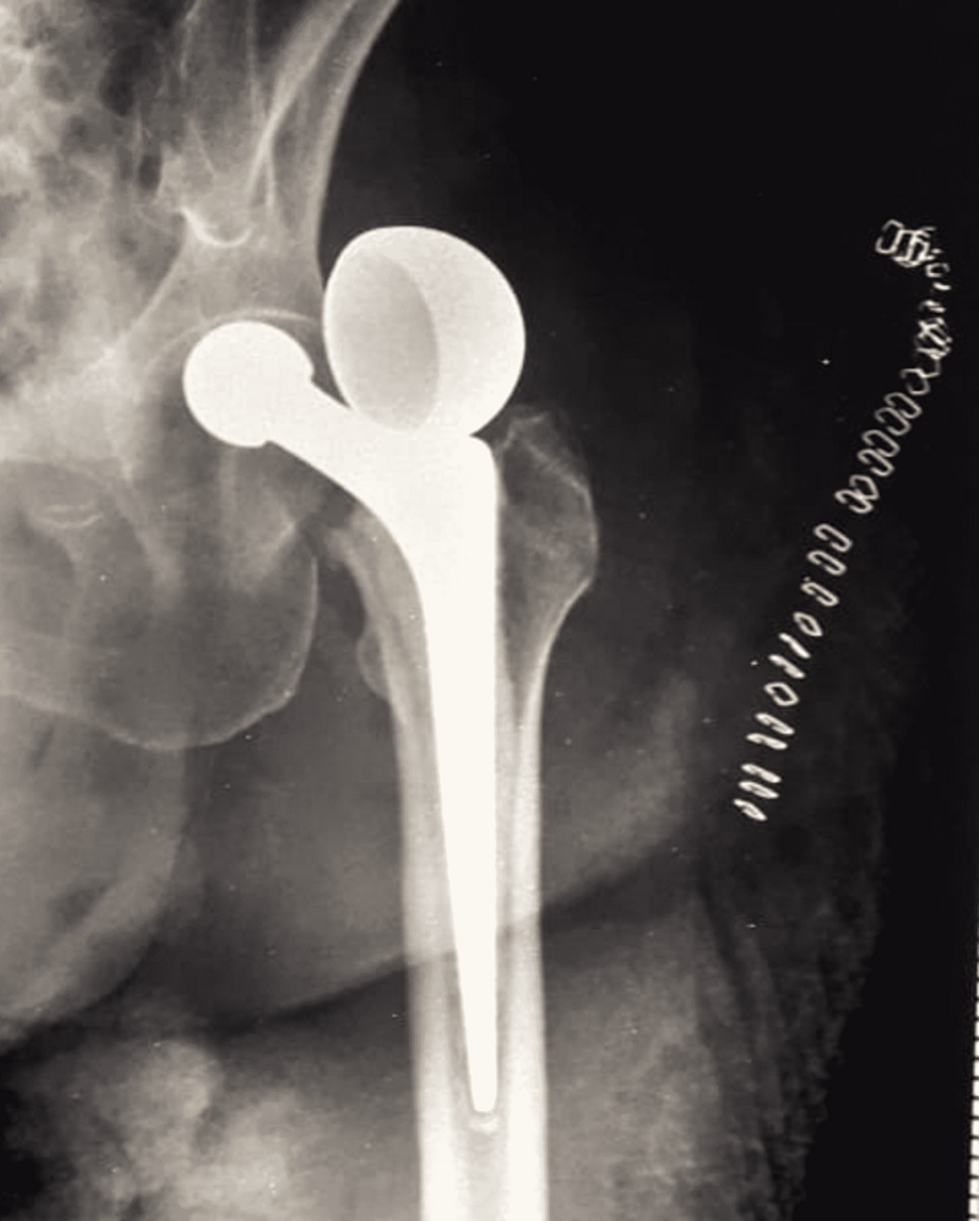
X-ray of the left hip showing dislocation and uncoupling of the femoral head X-ray shows the decoupled bipolar head lying in the supracetabular area.
